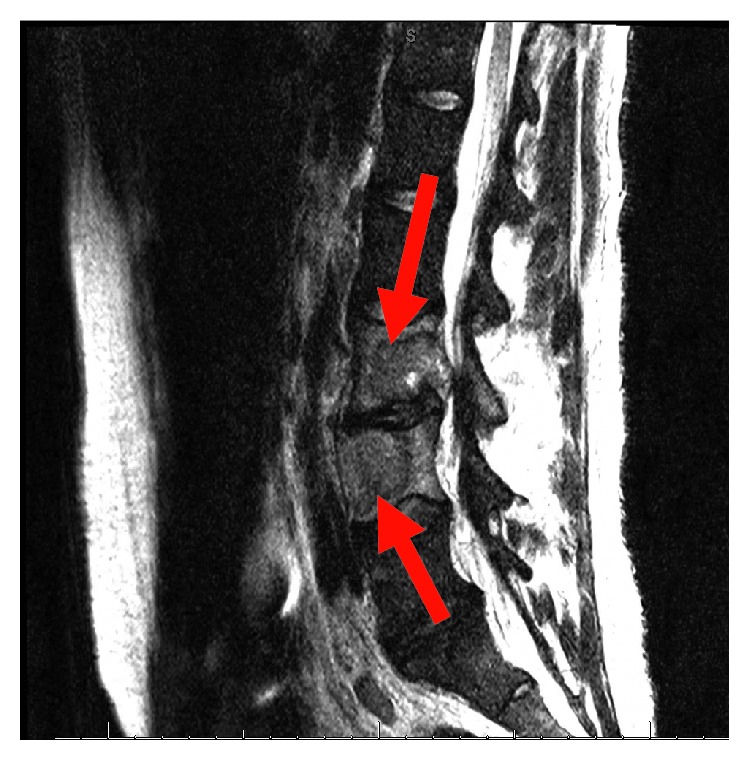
T2 sagittal MRI lumbar spine.
